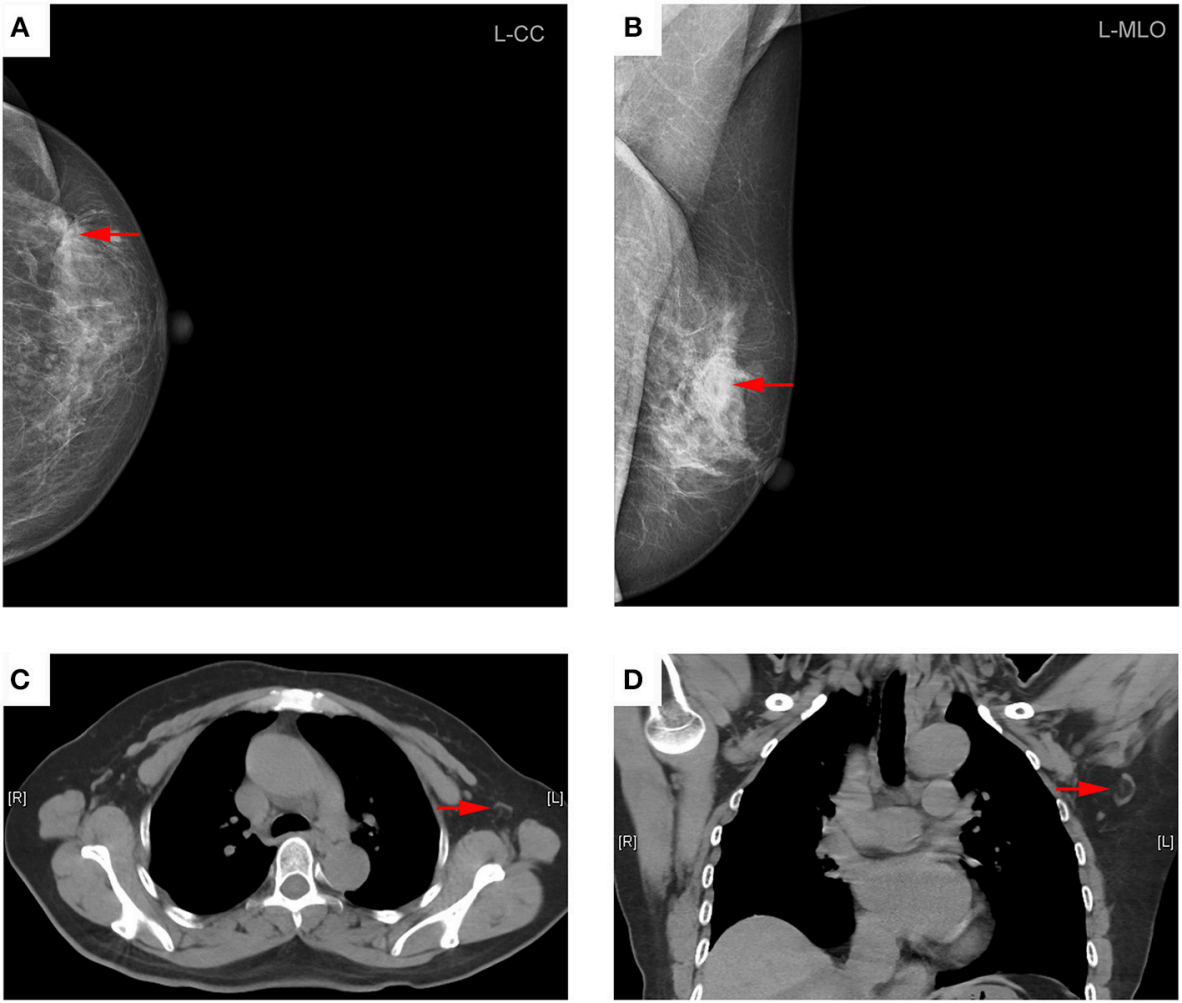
Representative mammography and computed tomography (CT) images of a 60-year-old woman with left primary breast cancer (stage pTisN0, DCIS). (A) mammography of craniocaudal image showed a mass in the lateral of the left breast, irregular, and slightly high-density shadows (red arrows). (B) Mammography of mediolateral oblique images showed a mass in the upper quadrant of the left breast, irregular, and slightly high-density shadows, about 21 × 13 mm in size, with rough edges and spicule sign (red arrows). (C) Transverse CT image showed an axillary lymph node with oval shape and presence of fatty hilum, a cortical thickness about 2 mm (red arrows). (D) Coronal CT image showed this lymph node with oval shape and presence of fatty hilum, a long-axis diameter of about 18 mm, and a short-axis diameter of about 8 mm (red arrows). The patient underwent sentinel lymph node biopsy, five lymph nodes were removed and proved to be pathologic negative.
